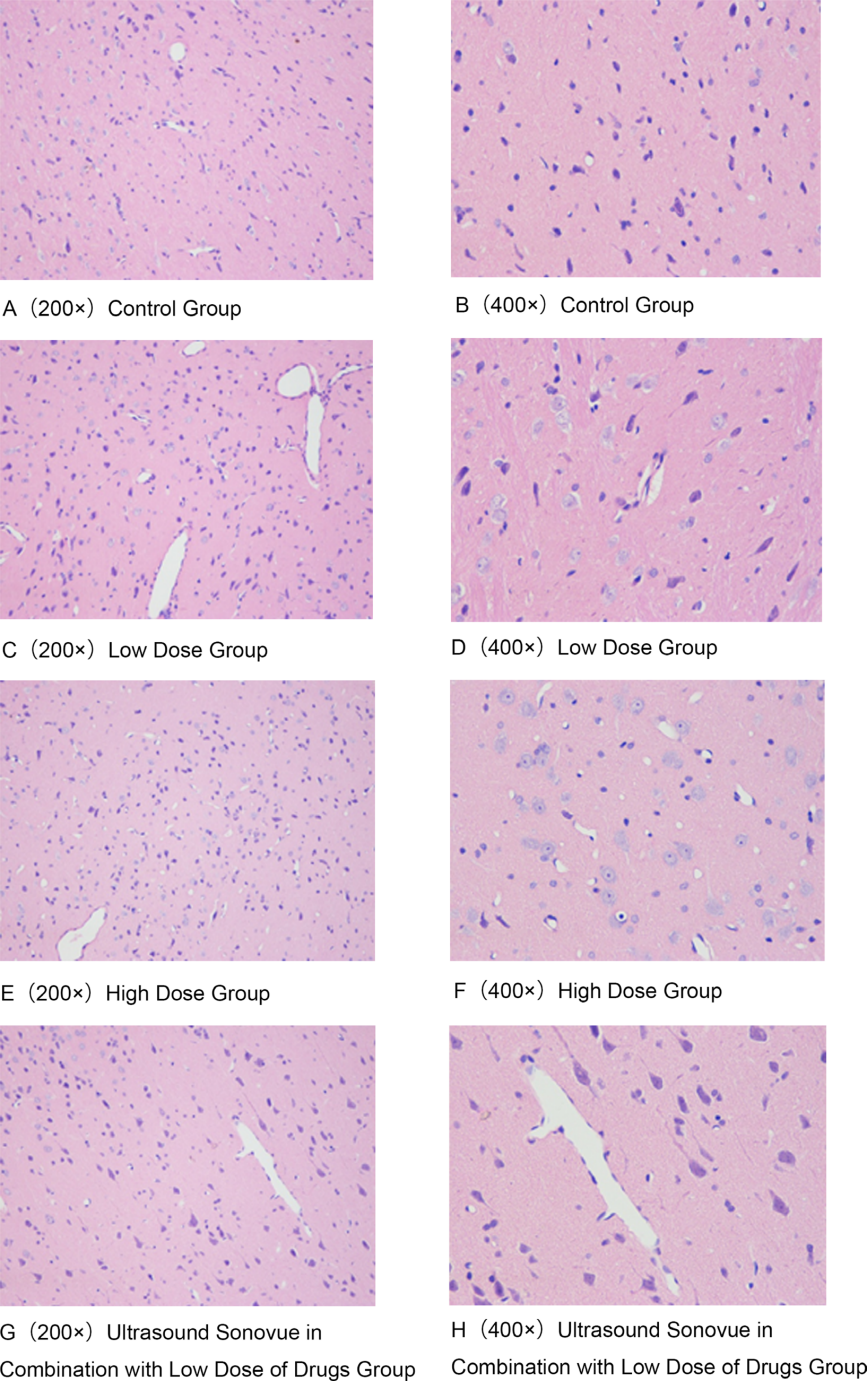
Ultrasound Sonovue^®^ combination of Cytarabine on brain HE dyeing results (Different groups indicated the RBC seepage situation has no difference.) (A) Blank Group 200×; (B) Blank Group 400×; (C) Low Dose Group 200×; (D) Low Dose Group 400×; (E) High Dose Group200×; (F) High Dose Group 400×; (G) Ultrasound Sonovue^®^ combined with Low Dose Drug Group 200×; (H) Ultrasound Sonovue^®^ combined with Low Dose Drug Group 400×.
